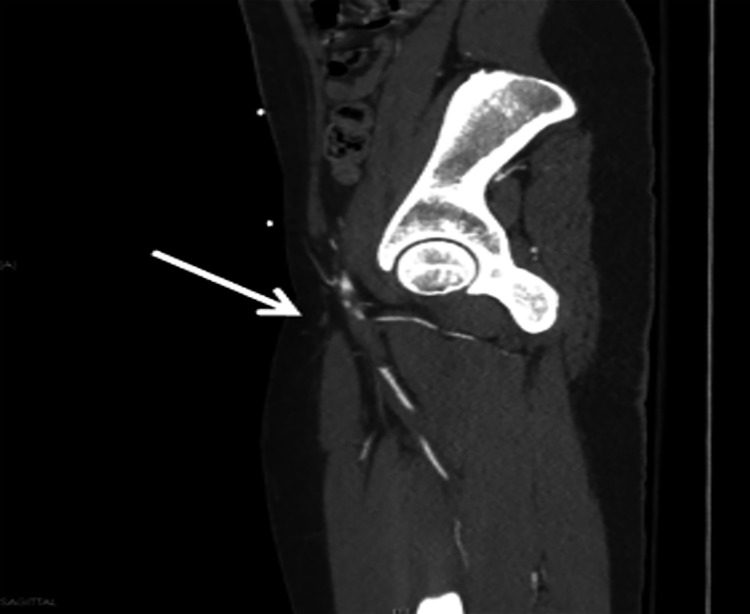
CT angiogram of the left lower extremity demonstrating thrombus with near-complete occlusion of the CFA (arrow). CFA, common femoral artery; CT computed tomography
